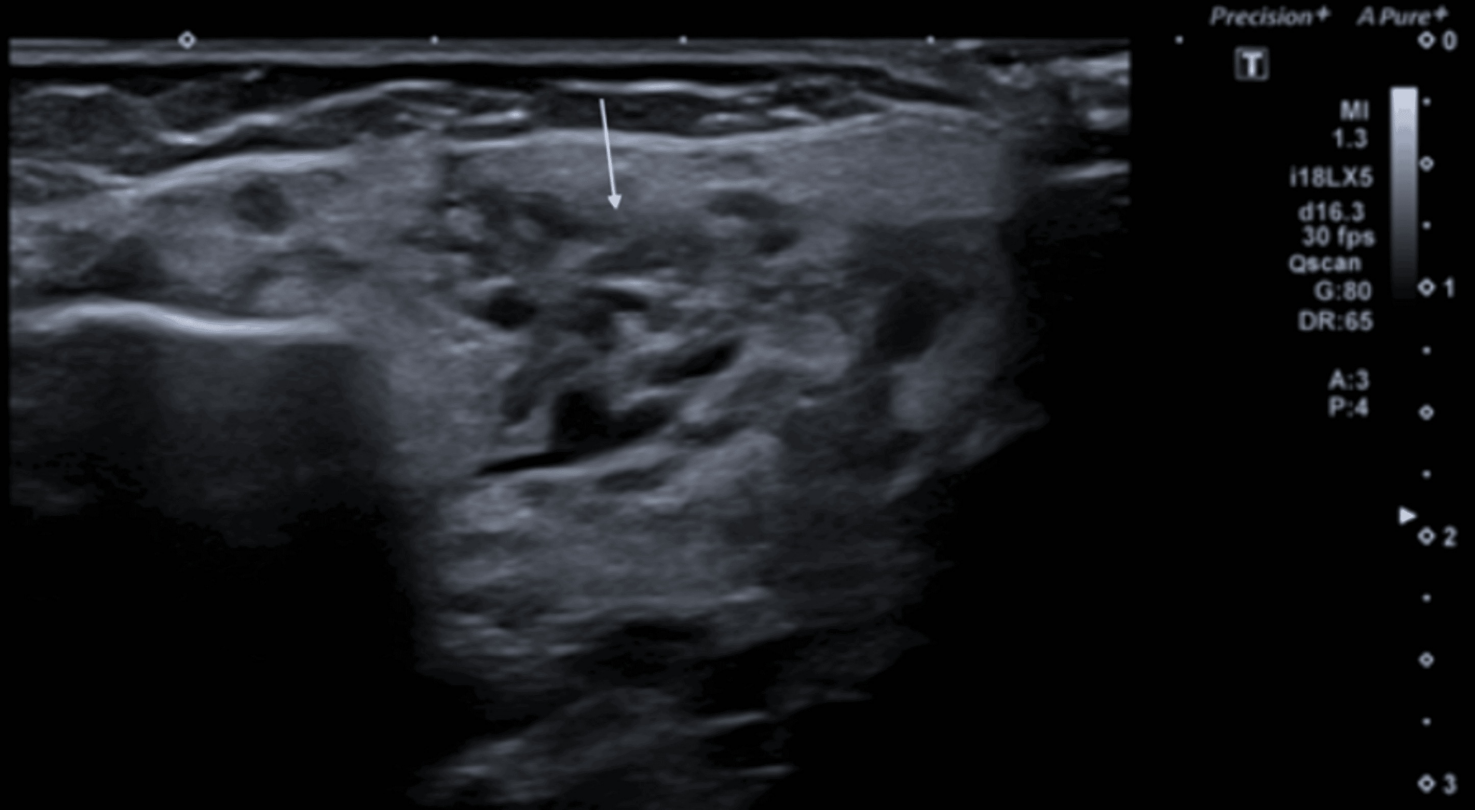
Follow-up ultrasounds demonstrated a good response, with complete regression of the parotid mass. The presence of a residual amorphous hypoechogenic area (white arrow) in the parotid gland is indicative of a residual lesion.
